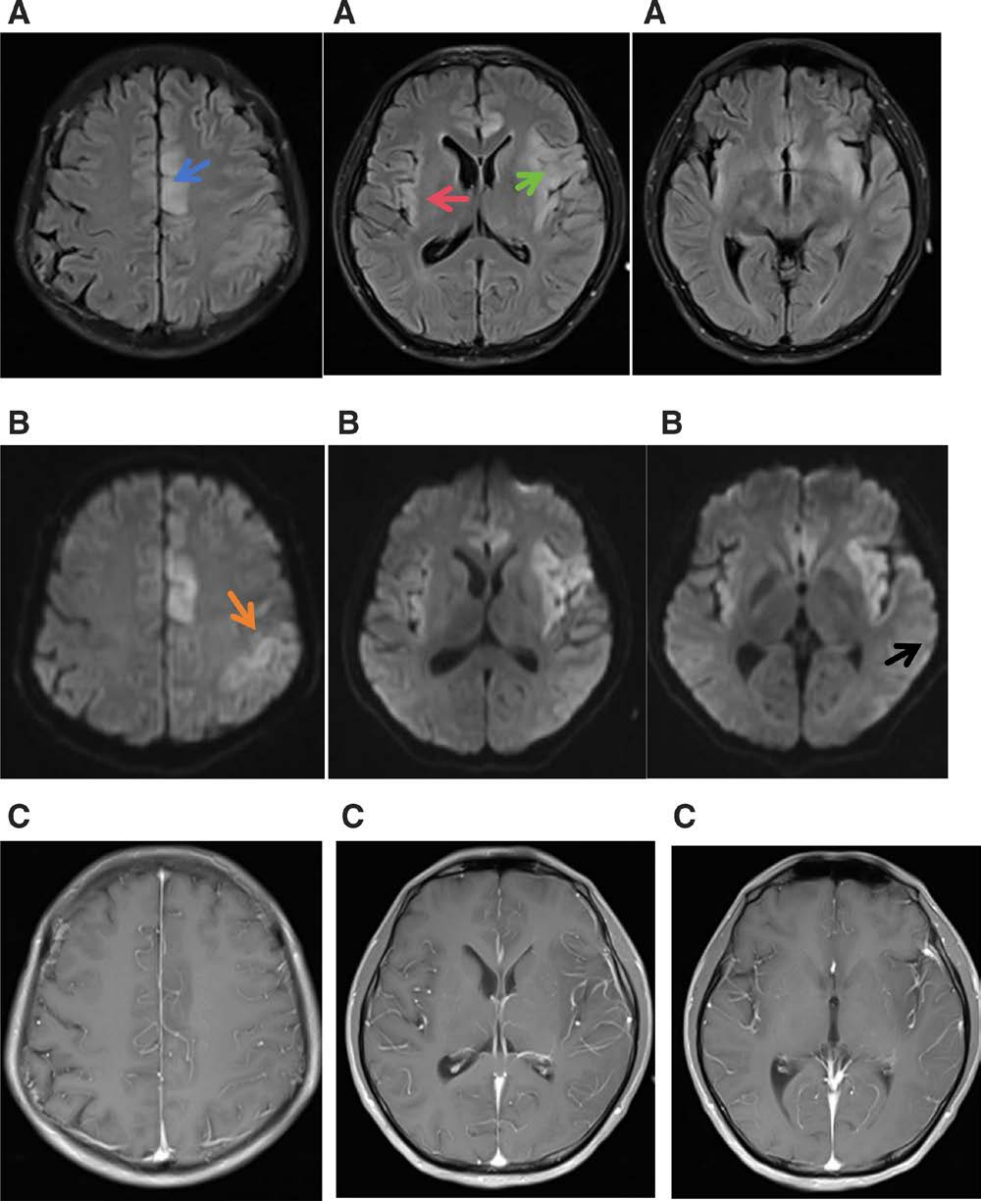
Cranial magnetic resonance imaging (MRI) showing T2 FLAIR, DWI, and enhanced T1 hyperintensity in the left frontotemporal, parietal, and occipital cortex and bilateral insula and the left cingulate gyrus. The lesion mainly involved the gray matter structure of the cortex. An enhanced scan shows meningeal enhancement. The blue arrow in (A1) indicates the FLAIR high signal in the left cingulate gyrus area. In (A2), the red arrow indicates FLAIR high signals in the right insula, while the green arrow indicates FLAIR high signals in the left insula and left frontotemporal lobe. The high DWI signal indicated by the orange arrow in (B1) is the left parietal lobe, and the high DWI signal indicated by the black arrow in (B3) is the right occipital cortex. DWI = diffusion weighted imaging, FLAIR = fluid attenuated inversion recovery, MRI = magnetic resonance imaging.
